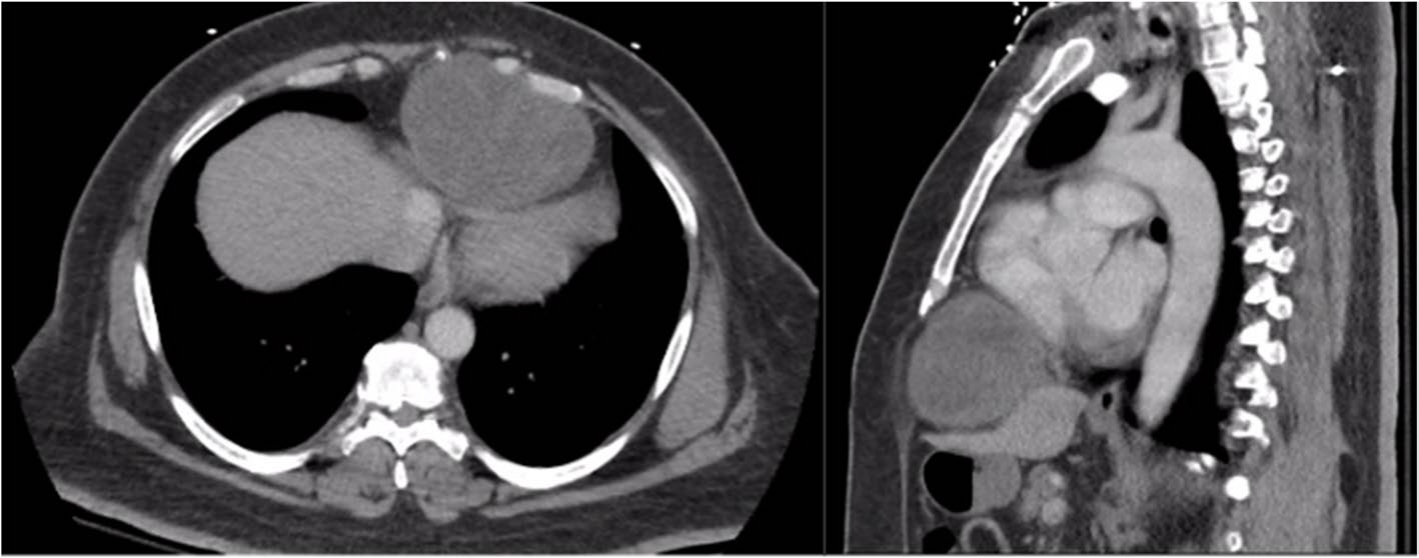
CT axial(left) and saggitial(right) chest and abdomen showing a large (10.6 x 7.2 x 8.3 cm) heterogeneous soft tissue mass in the mid/left paracentral region of the anterior lower thorax which abutted and caused mass effect upon the heart and diaphragm and partially extrudes at the level of the subxiphoid process
